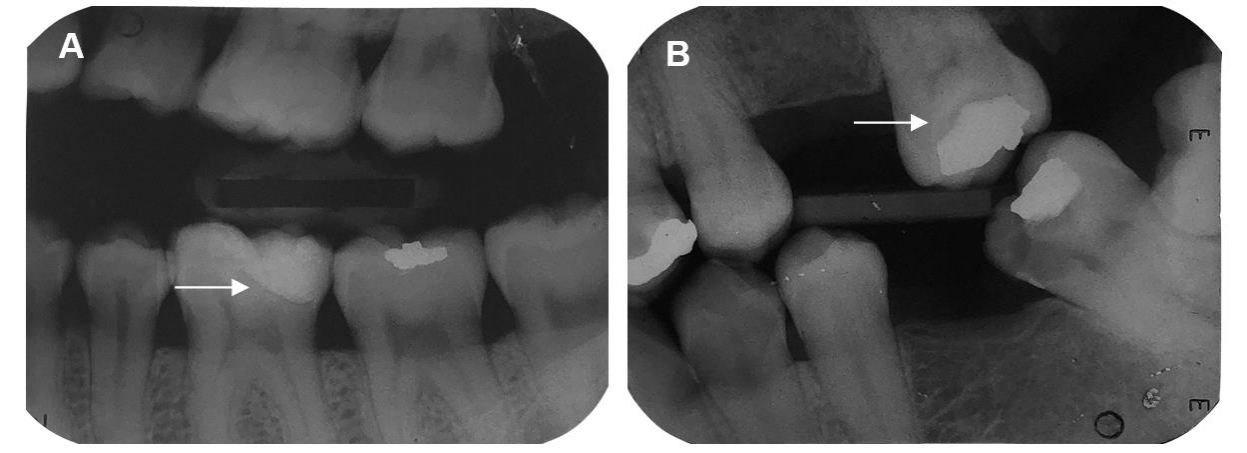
Examples of bitewing radiographs showing the presence of radiolucency incompatible with lesion around restoration. A: White arrow showing radiolucency line around the restoration compatible with an adhesive layer. B: White arrow showing radiolucency below the restoration compatible with residual caries issue after selective caries removal.
